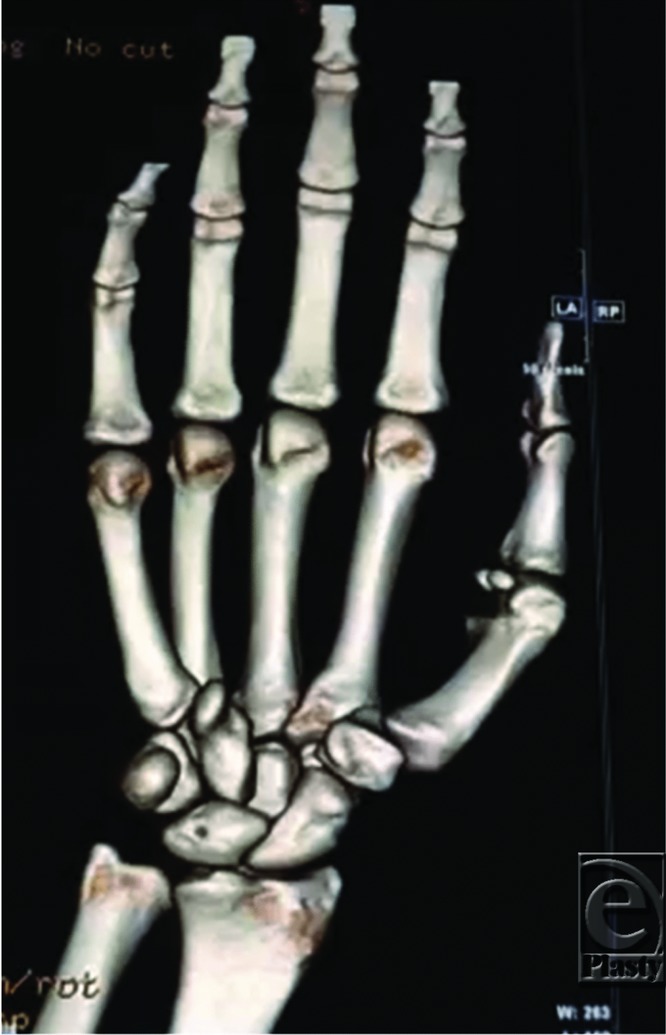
Right wrist 3-dimentional computed tomographic scan demonstration of dorsal perilunate dislocation, and clear evidence of dorsal dislocation of the fourth carpormetacarpal (CMC) joint and subluxation at the fifth CMC joint, as well as dislocation of the scaphoid/trapezium articulation and subluxation at the trapezium/trapezoid articulation.
